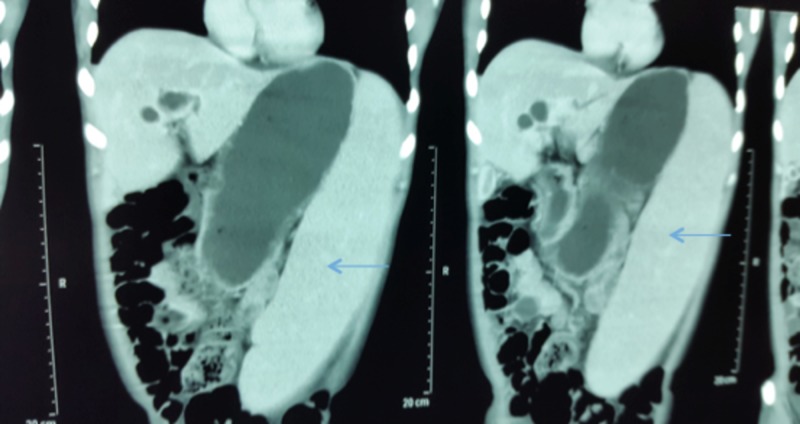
Computed tomography of the abdomen showing splenic enlargement (blue arrow)
